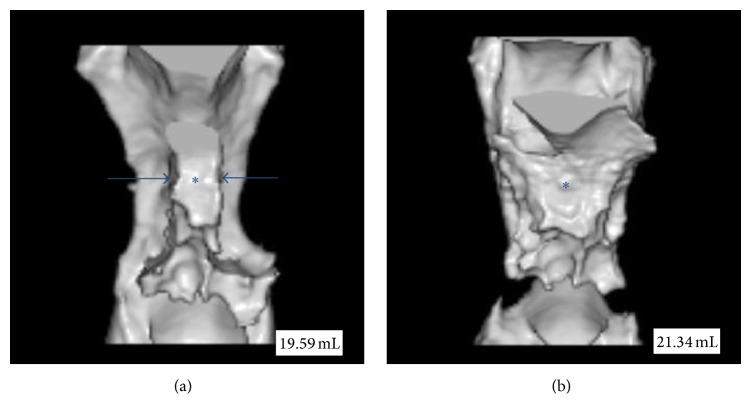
Anterior-view three-dimensional computed tomography images from patient 1. (a) Bilateral large tonsils impressed on the glossopharyngeal airway from both sides (blue arrows) before uvulopalatopharyngoplasty (UPPP). The gap between two arrows shows the airway (asterisk). (b) The airway was enlarged after UPPP. Nevertheless, the change in the volume of the airway (indicated by the number) was negligible.
